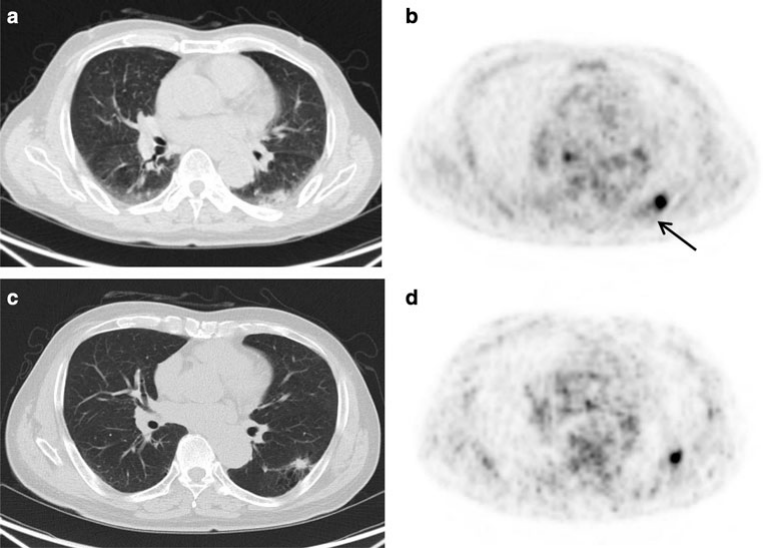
A 76-year-old male patient with a pulmonary lesion in the left lower lobe. RG CT (a), RG PET (b), DIBH CT (c), and DIBH PET (d). The RG PET SUVmax was increased by the poorly inflated dorsal lung with relatively high radioactivity (*arrow*)
